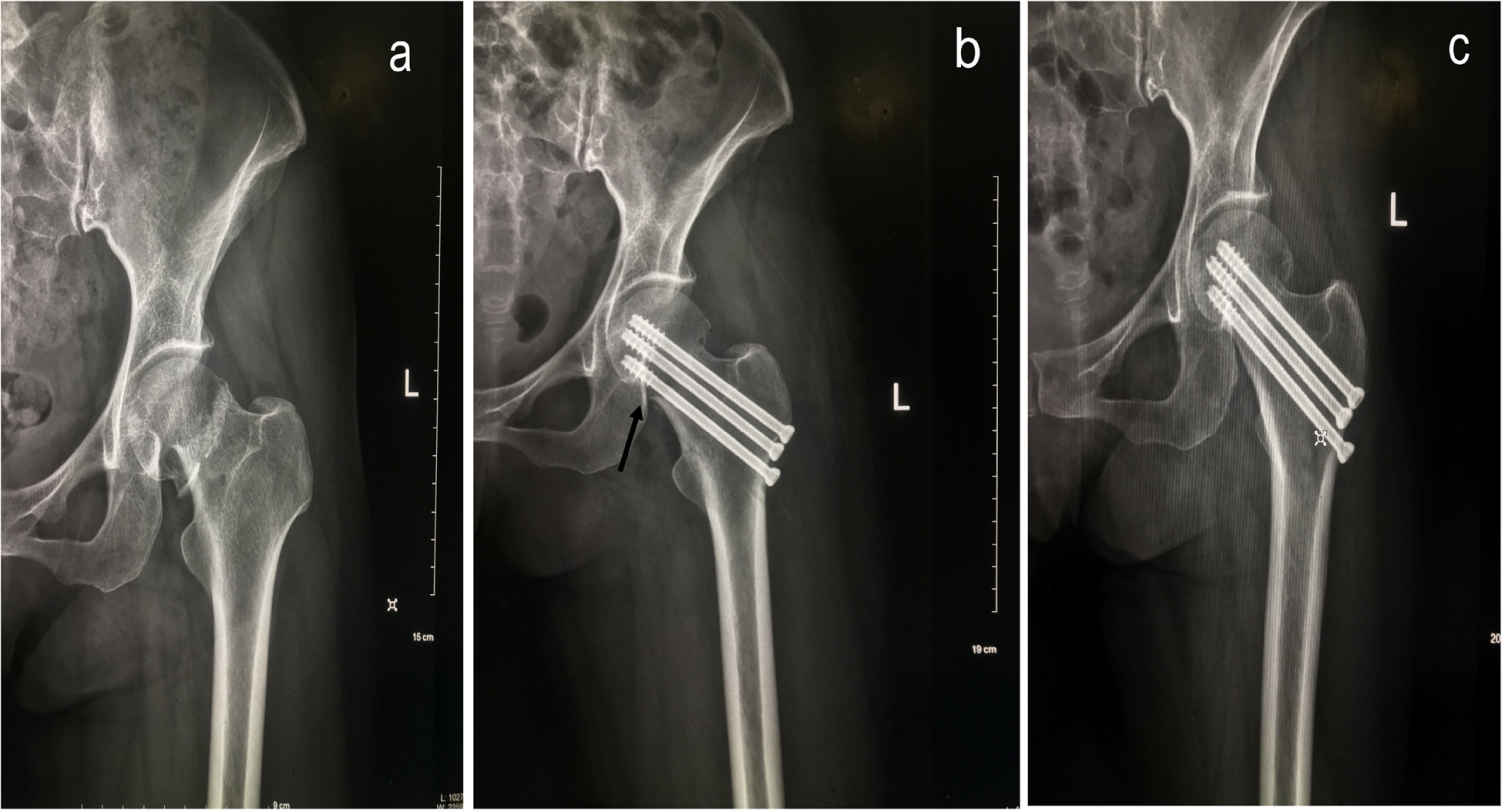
a Preoperative radiography. b Postoperative radiography and the arrow indicate positive buttress. c After a 2-year follow-up, radiography shows good fracture union without malunion
